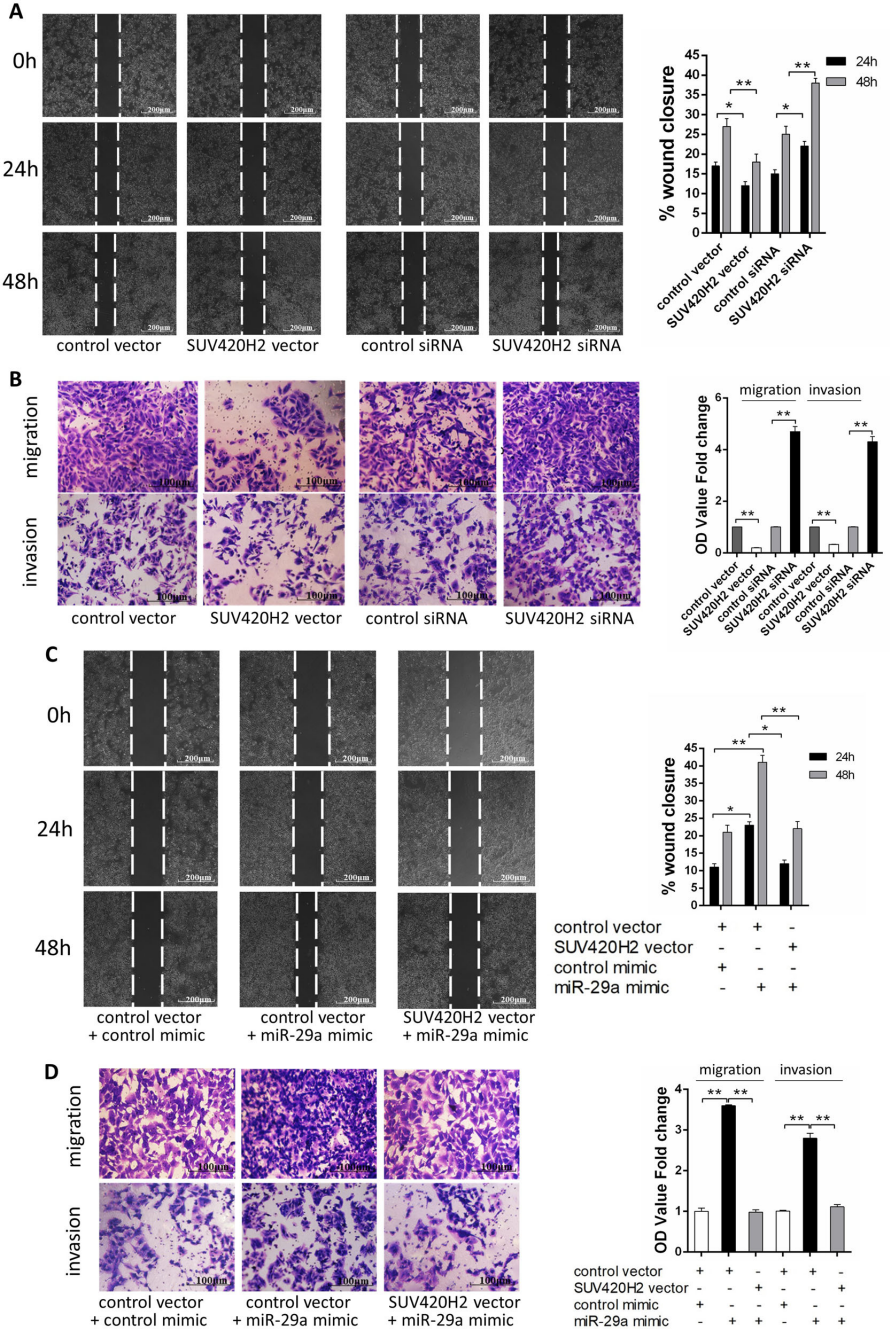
miR-29a promotes breast cancer cells migration and invasion via targeting SUV420H2. a, b Migration and invasion of MCF-7 cells transfected with either the control vector, SUV420H2 vector, control siRNA, or SUV420H2 siRNA as indicated, detected by wound healing assay (a), transwell migration assay, and transwell invasion assay (b). c, d Migration and invasion of MCF-7 cells transfected with either the control mimic plus control vector, miR-29a mimic plus control vector or miR-29a mimic plus SUV420H2 vector detected by wound healing assay (c), transwell migration assay and transwell invasion assay (d). P* < 0.05; *P* < 0.01
